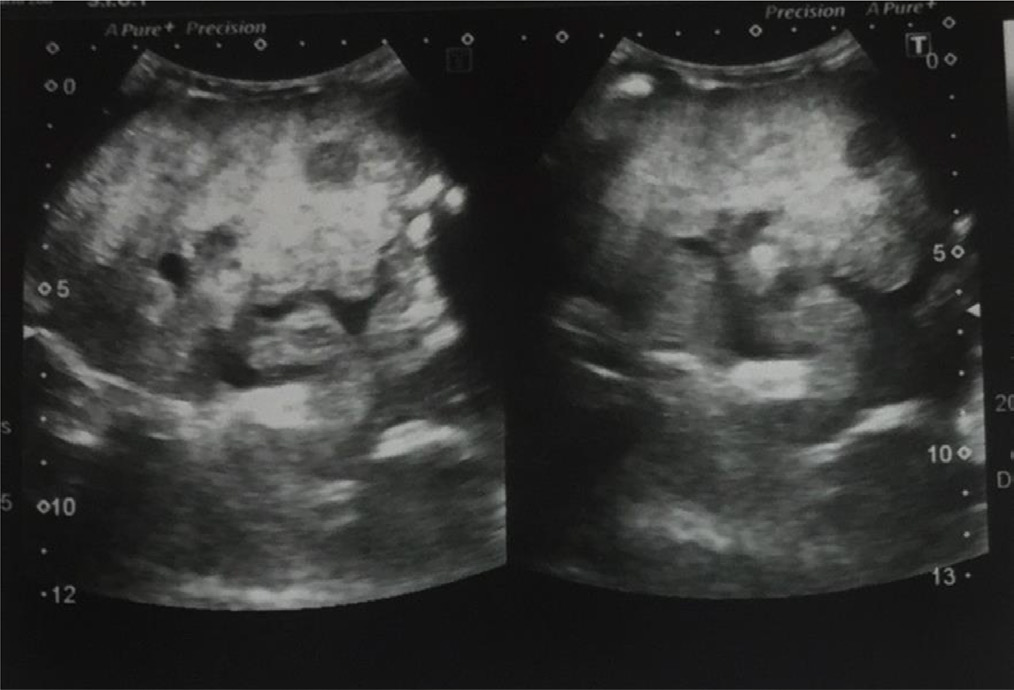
Ultrasound abdomen shows cirrhotic liver with irregular nodular margins and coarse texture. A small hypoechoic mass lesion is also evident within it.
